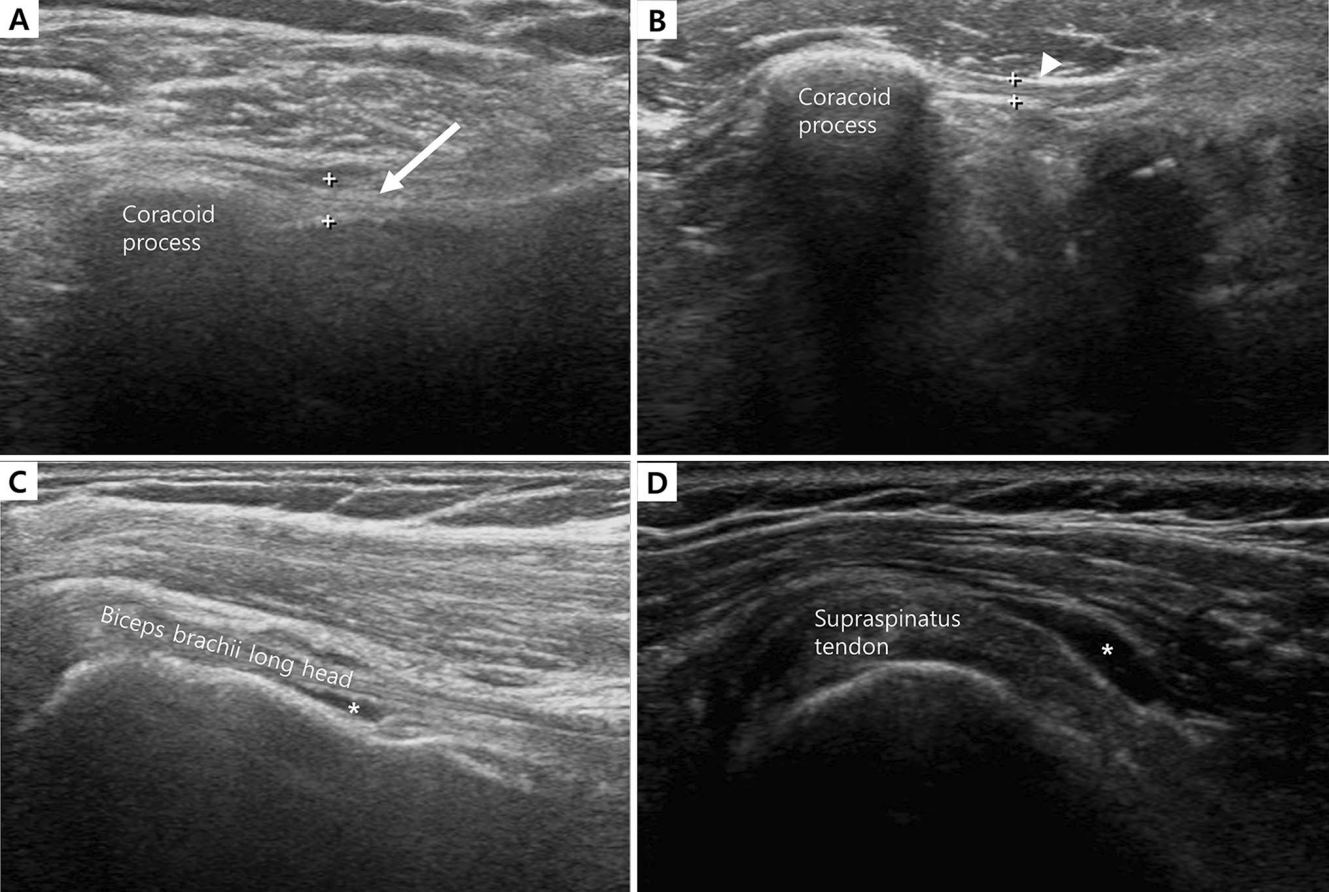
Representative image of shoulder ultrasonography in patients with chronic shoulder pain after breast cancer surgery. (A) Increased thickness of coracohumeral ligament (white arrow) in painful shoulder. (B) Normal thickness in contralateral side. (C) Peritendinous effusion (asterix) indicating biceps tenosynovitis. (D) Fluid collection (asterix) at subacromial-subdeltoid (SASD) bursa.
